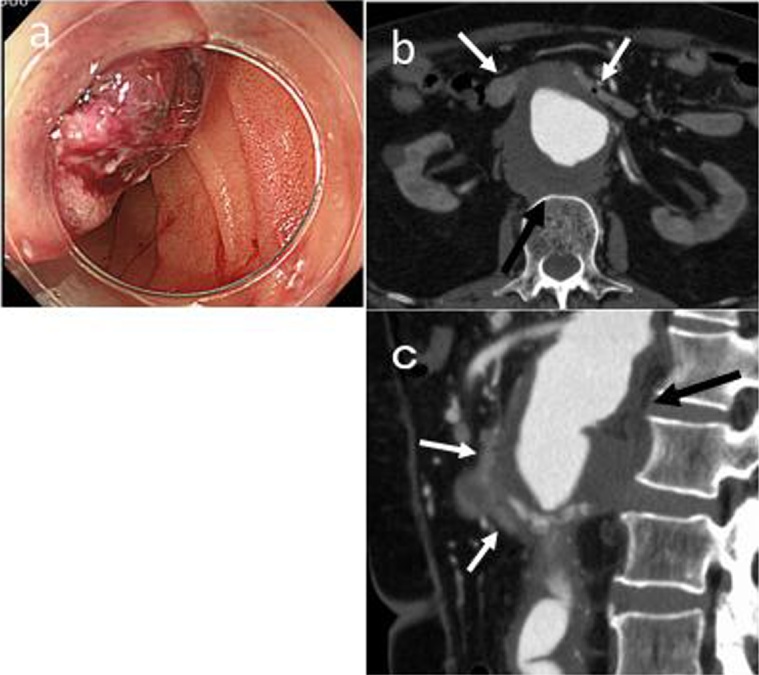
Upper gastrointestinal endoscopy (UGE)　and abdominal computed tomography (CT) scan. (a) UGE revealed the beating hematoma on the anal side of the major papilla. Axial (b) and sagittal (c) abdominal CT scan revealed the dilated irregularly shaped aneurysm (black arrow) on the proximal side of the prosthetic vascular graft, which compressed the duodenum (white arrows).
